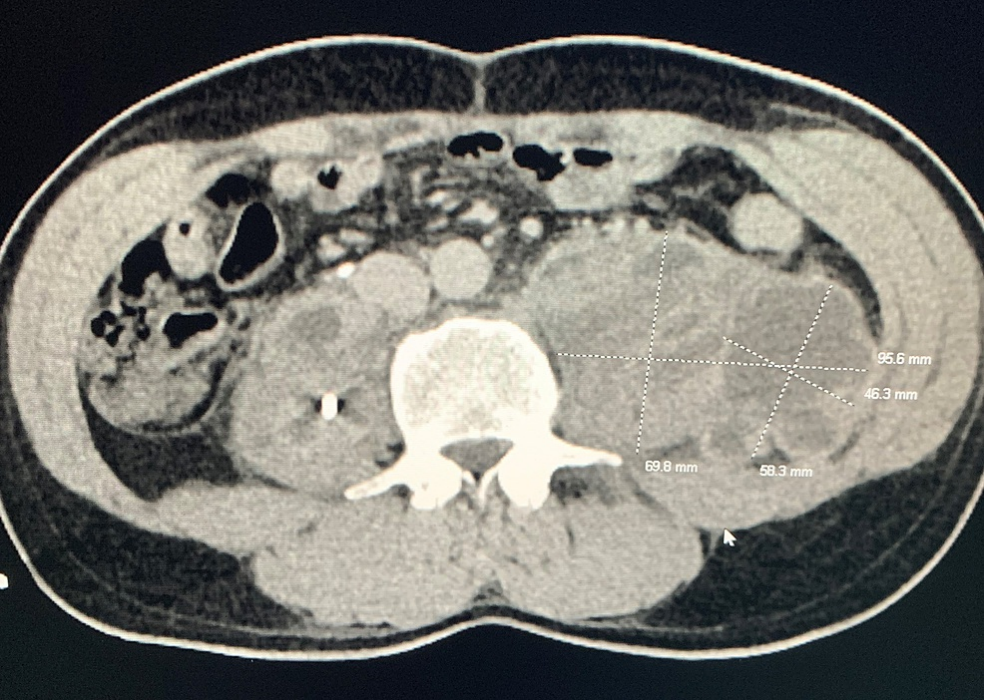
Axial CT demonstrating psoas abscesses with measurements Axial image of the abdomen at the level of the psoas muscles utilizing soft tissue windows. This demonstrates left greater than right psoas intramuscular complicated collections with thick intrinsic septations. There is a percutaneous drain present within the right psoas collection.
